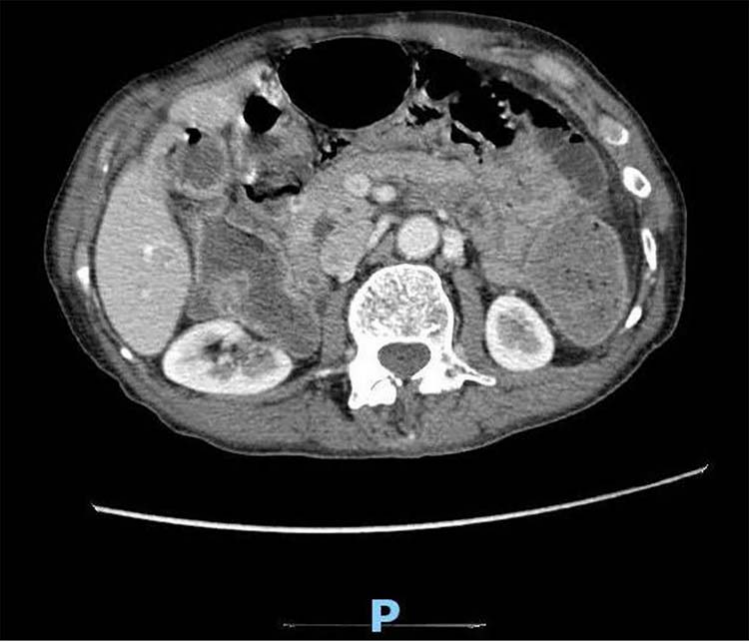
Enhanced CT scan of the abdomen showing evidence of partial large bowel with pneumobilia, gallbladder seen with interrupted wall and suspected fistula with the hepatic flexure (axial view).
